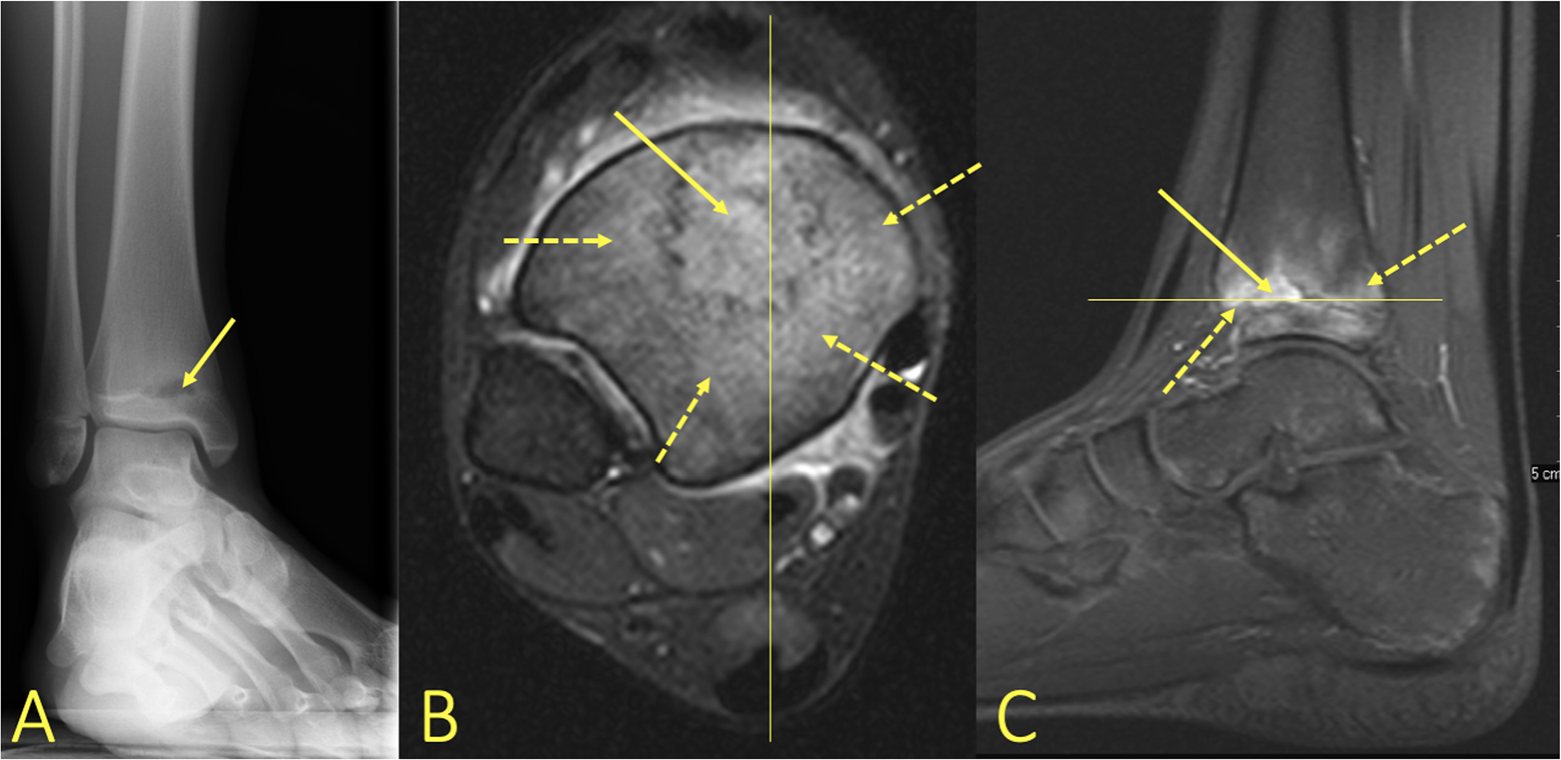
A 13-year-old female with intermittent moderate ankle pain for 3 weeks. a Radiographs showed a well-defined radiolucent lesion centrally in the distal tibial metaphysis (arrow). b, c MRI showed a Brodie abscess (arrow) with BME (dashed arrows) and contrast enhancement in distal tibia (arrow)
